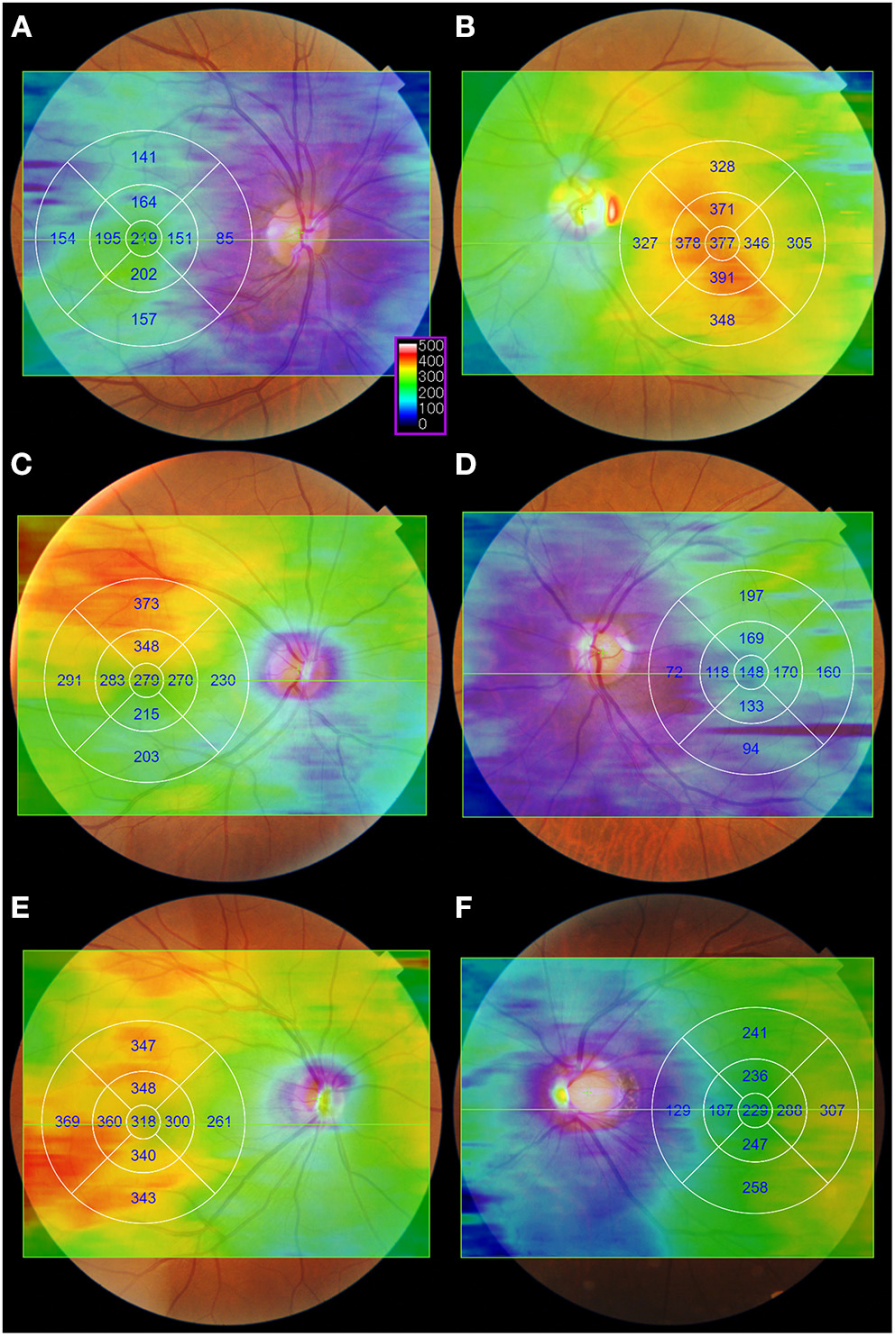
Examples of the color-coded choroidal thickness (CT) map in healthy eyes. The CT maps were generated automatically from a 12 × 9 mm three-dimensional volume scan consisting of a 256 B-scan with 512 A-scans per B-scan. Images were obtained using swept-source optical coherence tomography system (DRI OCT Triton, software version 10.17.003.01; Topcon Corp., Tokyo, Japan). A color-coded CT map and early treatment diabetic retinopathy study (ETDRS) grid were superimposed on the fundus photography using the built-in software. After the automatic layer segmentation, a color-coded CT map was obtained with the Bruch's membrane and choroidoscleral interface selected as the inner and outer limits of segmentation lines, respectively. The color scale (purple box) shows the choroidal thickness in microns. On a color-coded CT map, the warmer colors represent the thicker areas, while the cooler colors represent the thinner regions. (A,B) Representative cases of eyes with the greatest CT at the fovea. (C,D) Representative cases of eyes with the greatest CT it the superotemporal region. (E) Representative case of eyes with the greatest CT it the inferotemporal region. (F) Representative cases of highly myopic eyes with the greatest CT in the temporal region.
